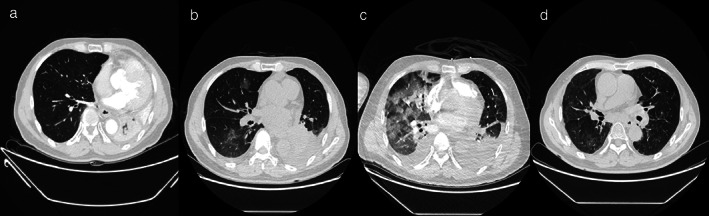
(a) CT scan shows left lung atelectasis. (b). CT scan shows multiple mild diffuse ground‐glass opacity (GGO) in the right lung after two days of crizotinib and osimertinib administration. (c) CT scan shows multiple severe diffuse GGO in both lungs after seven days of crizotinib and osimertinib treatment. (d) CT scan taken six weeks later shows diffuse GGO absorbed after glucocorticoid therapy.
